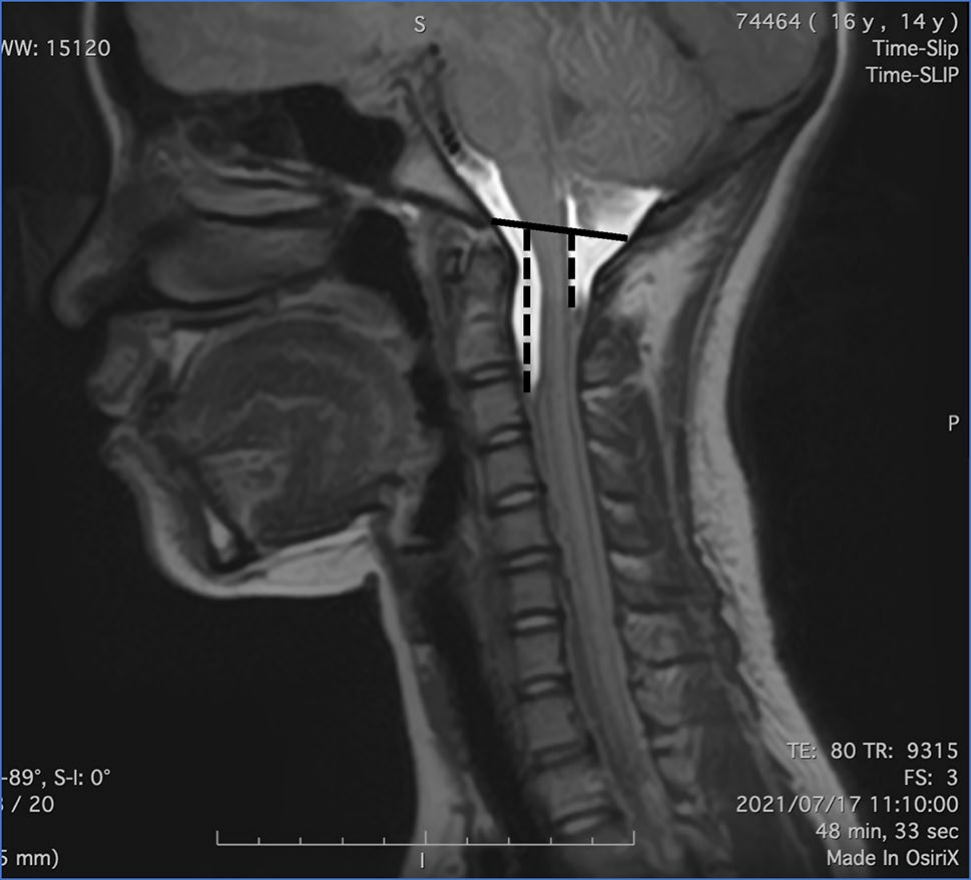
Travel distance measurement using Time-SLIP MRI. The solid line indicates the Bastion-opisthion line. The dotted lines indicate the maximum movement distances of CSF on ventral and dorsal side of the spinal canal. Travel distance was measured as the sum of the maximum movement distances of CSF on the ventral and dorsal sides of the spinal canal from Bastion-opisthion line within a single timeframe was defined and measured as TD.
